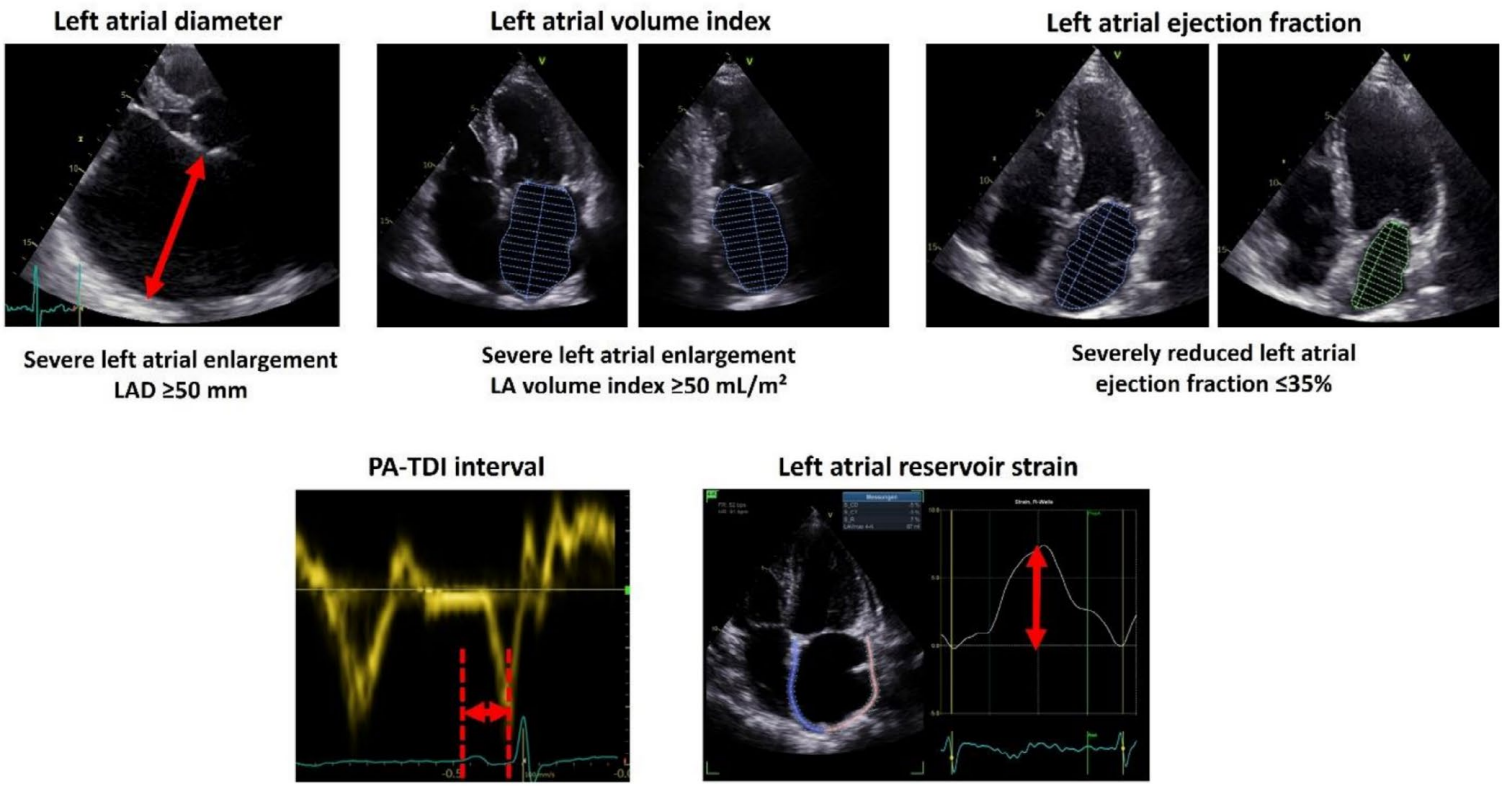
Echocardiographic parameters indicating atrial cardiomyopathy.
